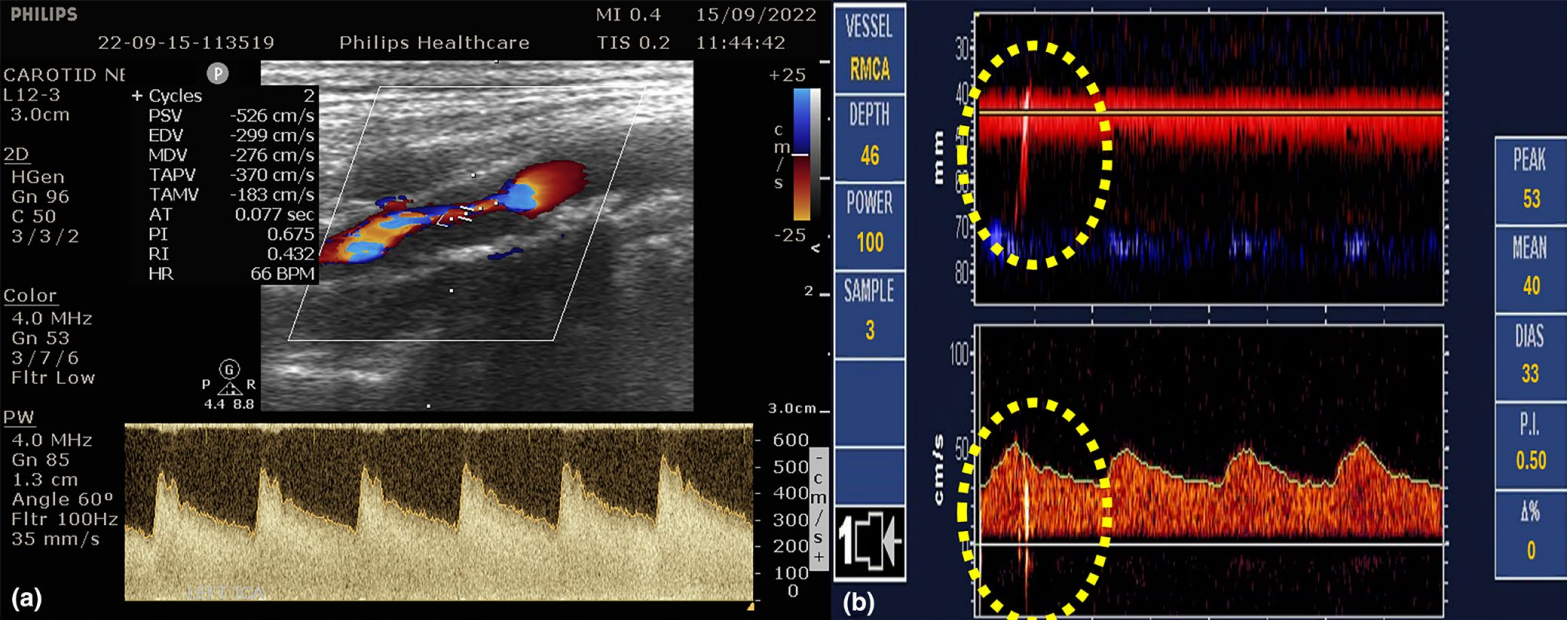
Patient with acute ischemic stroke due to symptomatic internal carotid artery stenosis, showing a hypoechoic plaque with a peak systolic velocity of more than 400 cm/sec causing >90% stenosis of the right internal carotid aartery (a) with positive microembolic detection on the ipsilateral middle cerebral artery as detected by Transcranial Power Motion‐Mode Doppler ((b) upper image) and in Doppler spectrum ((b) lower image).
